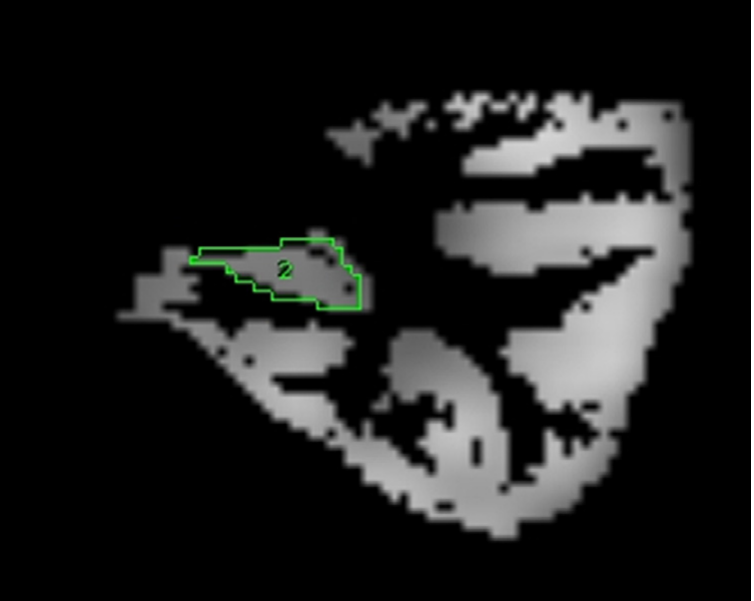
FDG-PET left temporal cortex with coregistered hippocampal tracing, coronal section.
